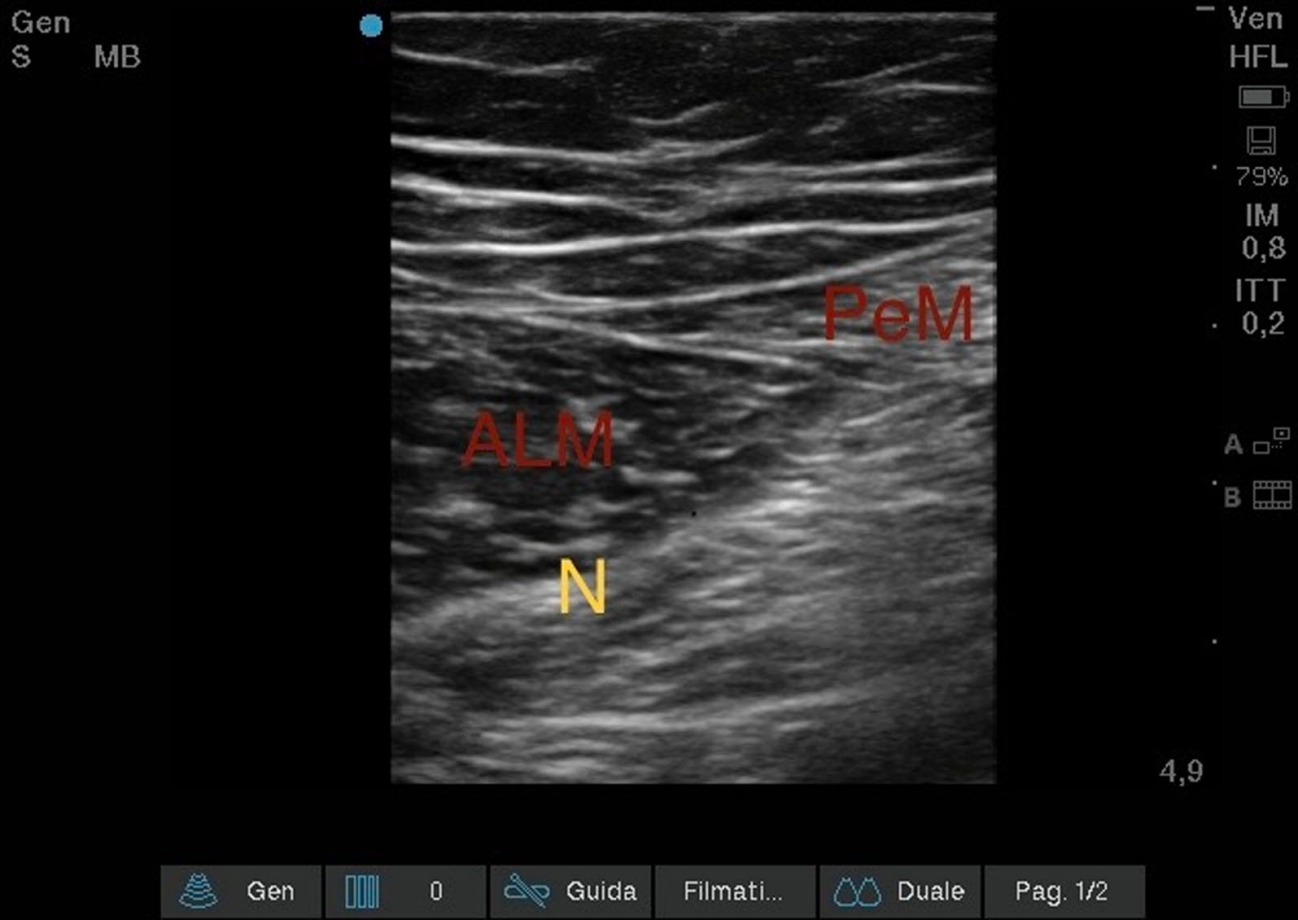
Ultrasound obturator nerve block. Adductor longus muscle (ALM); Pectineus muscle (PeM); Anterior branch of obturator nerve (N)
